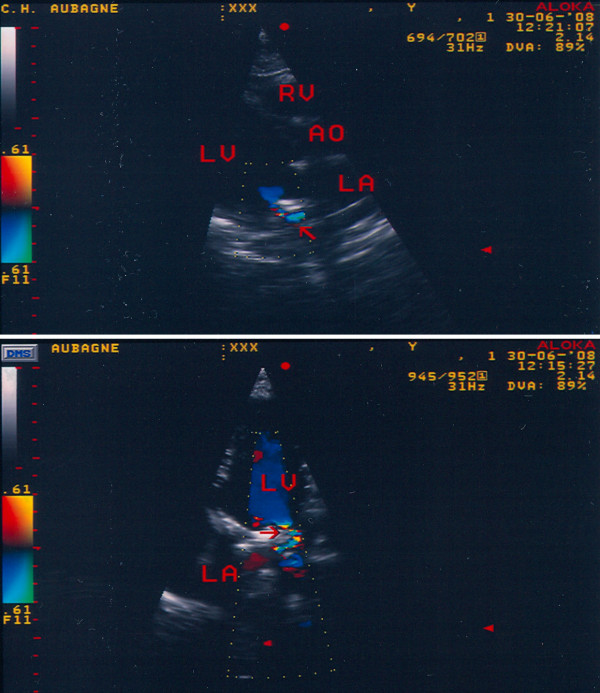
Visualization of the paravalvular prosthetic mitral regurgitation (arrow) in parasternal long axis view (upper part) and 4-chamber apical view (lower part). AO: aorta; LA: left atrium; LV: left ventricle; RV: right ventricle.
